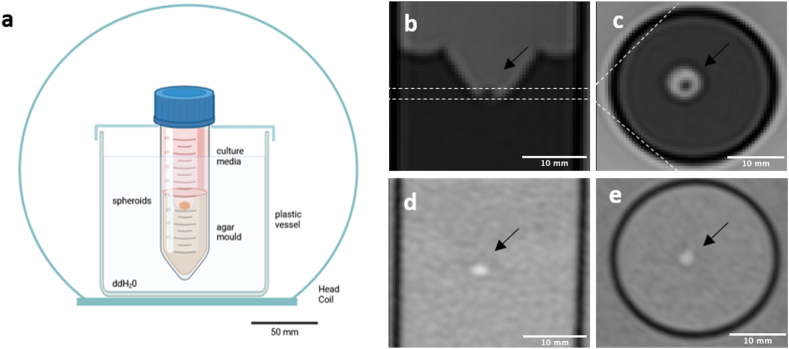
Visualization of SW1353 cell spheroids (n = 192 per tube, 62.500 cells per spheroid, indicated by arrow) in a mold of 1 % agar and culture media. Images were captured on the day of spheroid collection (day 0) to ensure immediate and accurate representation. The experimental setup was designed using Biorender.com (a), while sagittal (b) and coronal (c) T2-weighted images were obtained using an FSE sequence. Sagittal (d) and coronal (e) T1-weighted images were obtained using a spoiled gradient echo VIBE sequence. Black scale bar represents 50 mm, white scale bars 10 mm, respectively.
